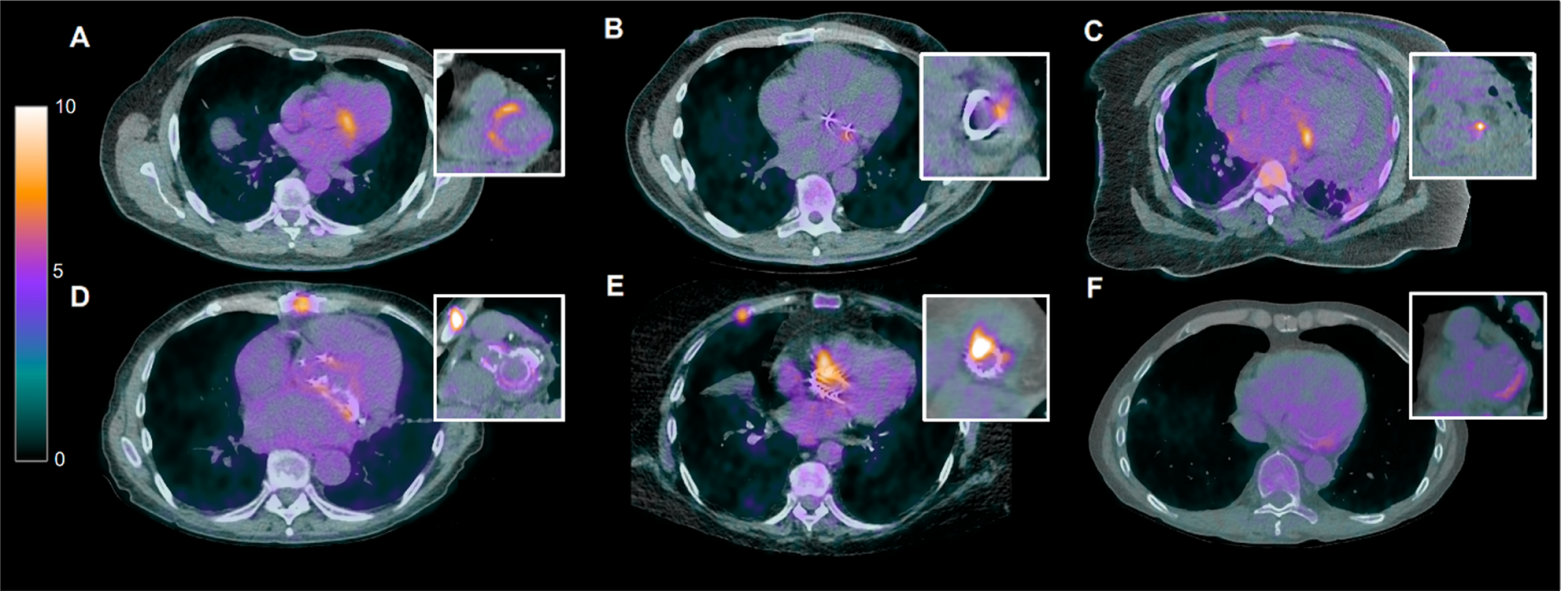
Representative images of Endocarditis on PET-CT. Images acquired following a high fat, low carbohydrate diet, 12-hour fast and intravenous heparin to achieve suppression of physiological myocardial uptake (myocardial suppression). Panel A NVE of mitral valve; Panel B PVE of a mitral valve ring; Panel C NVE of mitral valve with pericardial effusion; Panel D post-surgical uptake in mechanical aortic and mitral valve replacements; Panel E PVE with root abscess in TAVI; Panel F NVE of mitral valve. *NVE*, native valve endocarditis; *PET-CT*, ^18^Fluoride fluorodeoxyglucose position emission tomography with computed tomography; *PVE*, prosthetic valve endocarditis; *TAVI*, transcatheter aortic valve implant
